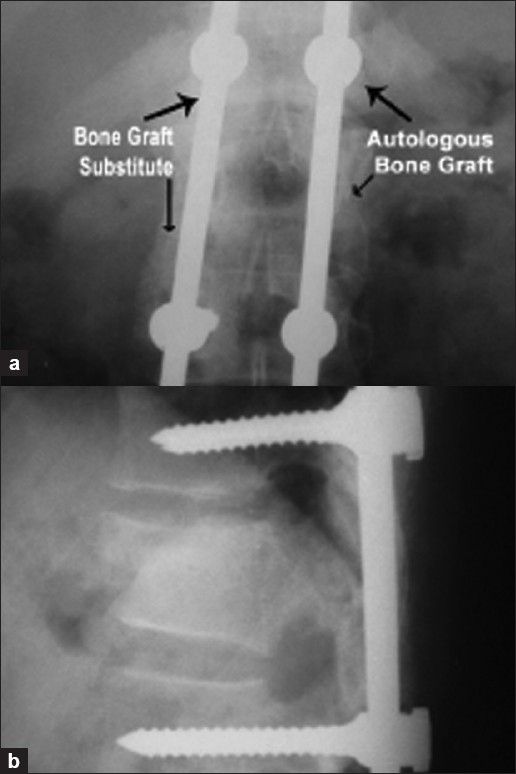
Six-month follow-up X-ray [anteroposterior view (a) and lateral view (b)] of the same patient showing pedicle screw and rod assembly *in situ* with fusion in progress on both the sides
